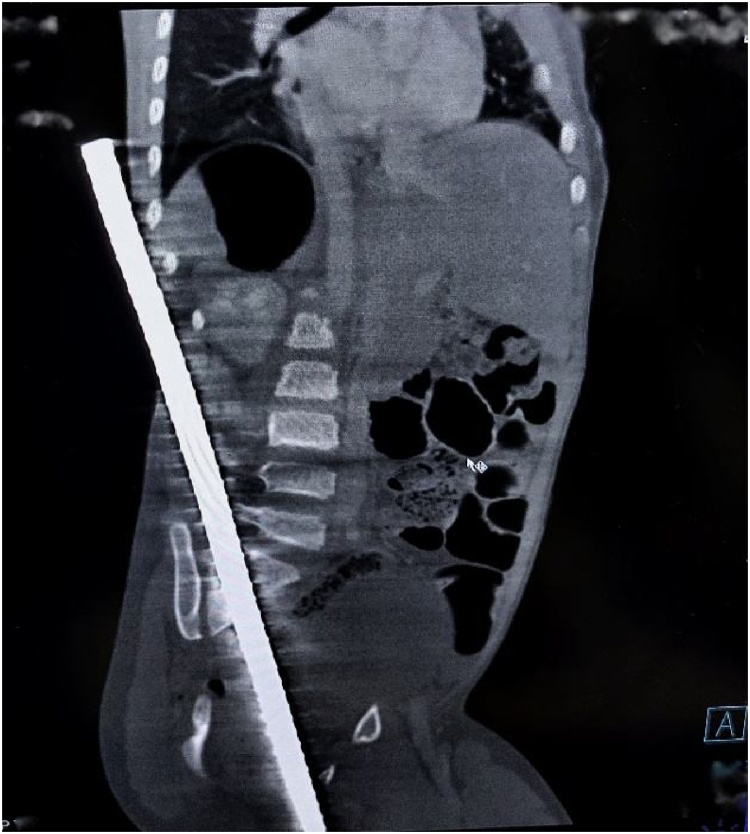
CT scan (oblique sagittal section) showing high density foreign body (rod) passing adjacent to S1 vertebrae from perineum to loin.
